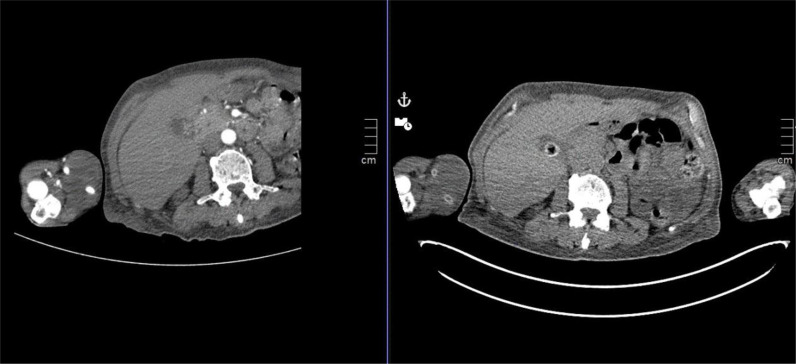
Computed tomography (CT) scan showing the mass. CT scan, axial view, and image with (left) and without (right) contrast of the right forearm and abdomen showing a recurrent non-enhancing soft tissue lesion at the antecubital fossa, measuring 6 cm in the craniocaudal dimension, and encasing the AVS without any definite vascular compromise nor evidence of associated bony destruction.
